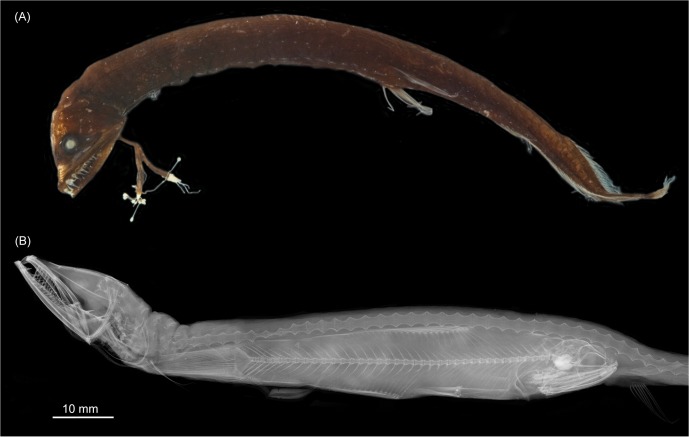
External and internal morphology of *Eustomias* spp. (A) *Eustomias fissibarbis*, USNM 270587. (B) X-ray of *Eustomias obscurus*, USNM 206711 showing a large, ingested myctophid.
